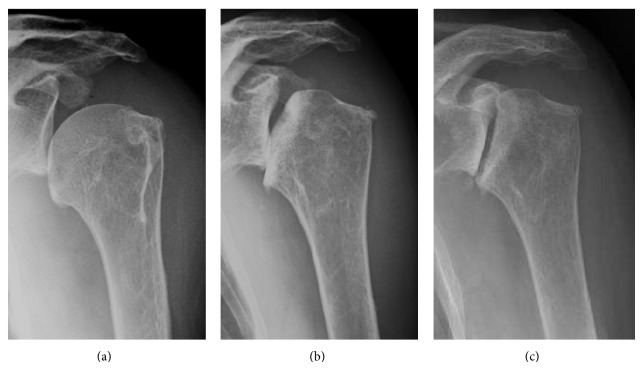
Case  2. Serial preoperative radiographs. (a) The humeral head is slightly displaced inferiorly, but the shape is maintained at the time of symptom onset. (b) Five months later, the humeral head demonstrates progressed collapse. (c) Bone defects of the glenoid are noted 2 years after symptom onset.
